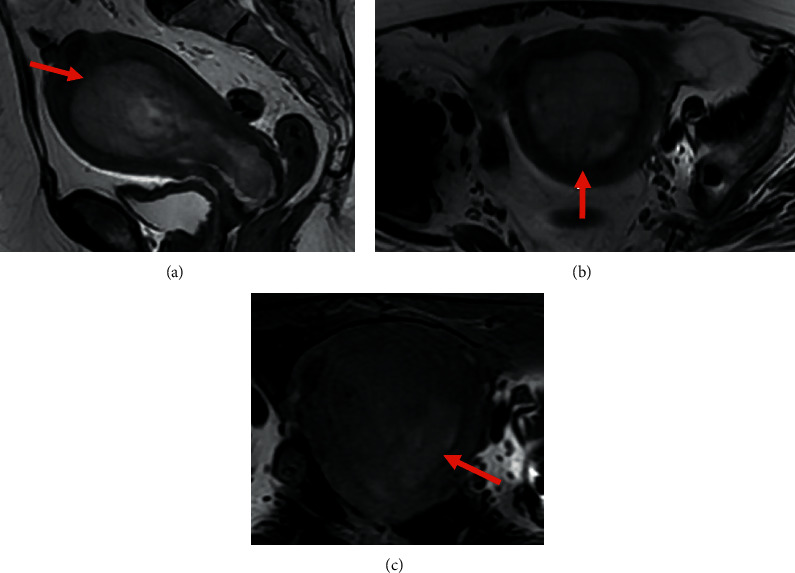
Pelvic MRI of endometrial stromal sarcoma: (a, b, c) sagittal (a) and axial (b) T2WI showing a large intrauterine tumor with heterogeneous intermediate T2 signal with invasion of junctional zone (arrow); and axial T1 Fat-Sat WI (c) showing high signal intensity intratumoral hemorrhage (arrow).
